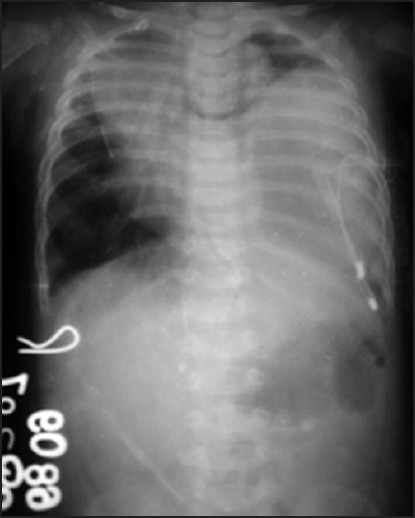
Same chest X-ray after ICT insertion
